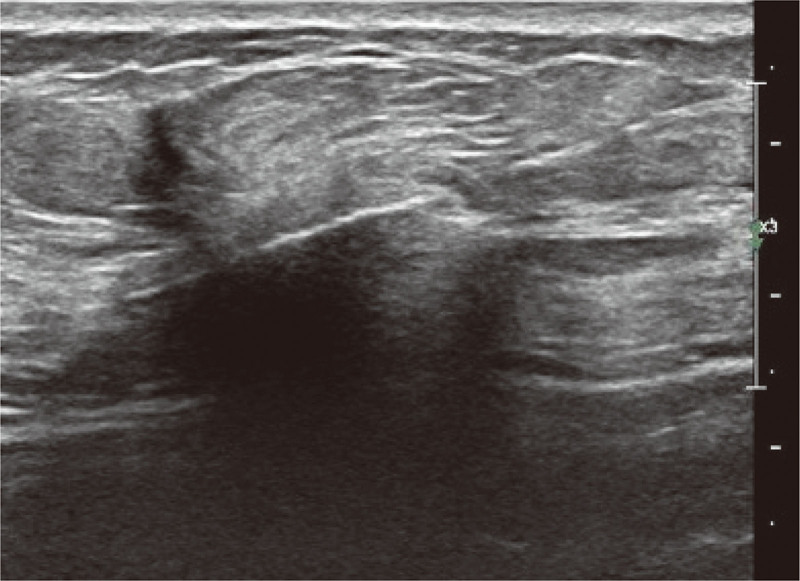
Ultrasound-guided puncture biopsy of breast tumors. CNB was performed under the guidance by Color Ultrasound Diagnostic Instrument (GE Healthcare, Boston, MA, USA). The frequency of the probe was 10 MHz. Magnum Disposable Core Tissue Biopsy Needle (Bard; BD Biosciences, Franklin Lakes, NJ, USA) had a needle groove length of 20 to 22 mm. CNB = core needle biopsy.
